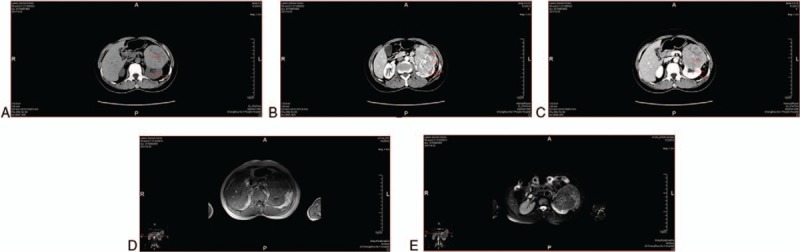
(A) A mixed density mass with a size of 7.9 × 7.6 cm and clear boundary in the lower left kidney were observed. Tumor attenuation (68 HU) was greater than renal parenchyma (33 HU) and medulla (28 HU) during CT plain scan. (B) and (C) There are slightly high and low density necrosis in the inferior plane of the tumor, revealing obvious uneven enhancement and the phenomenon contrast agent fast forward and fast out. The left renal pelvis oppressed by the tumor was unclear. After the peritoneum, the nodular shadow was seen in the retroperitoneal region, showing obvious inhomogeneous enhancement. The attenuation (98 HU in the arterial phase, 101 HU in the venous phase) was weaker than renal parenchyma (180 HU in the arterial phase, 202 HU in the venous phase) and medulla (142 HU in the arterial phase, 125 HU in the venous phase) in both arterial and venous phases. (D) and (E) The left kidney was with an irregular contour. In the upper left pole of the kidney, there were a mass of about 10.6 × 7.9 cm. This was mixed with a short T1 signal, and the internal signal was uneven. The vascular shadows and false envelop was observed in the tumor. Enlarged lymph nodes of about 2.3 cm were observed in the left renal hilar region. On MRI, the signal of the tumor was close to the renal cortex in the T1 and low signal intensity in T2.
